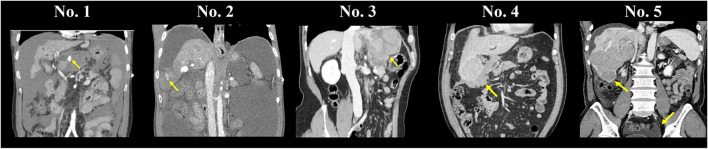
Computed tomography scan of a ruptured hepatocellular carcinoma.
